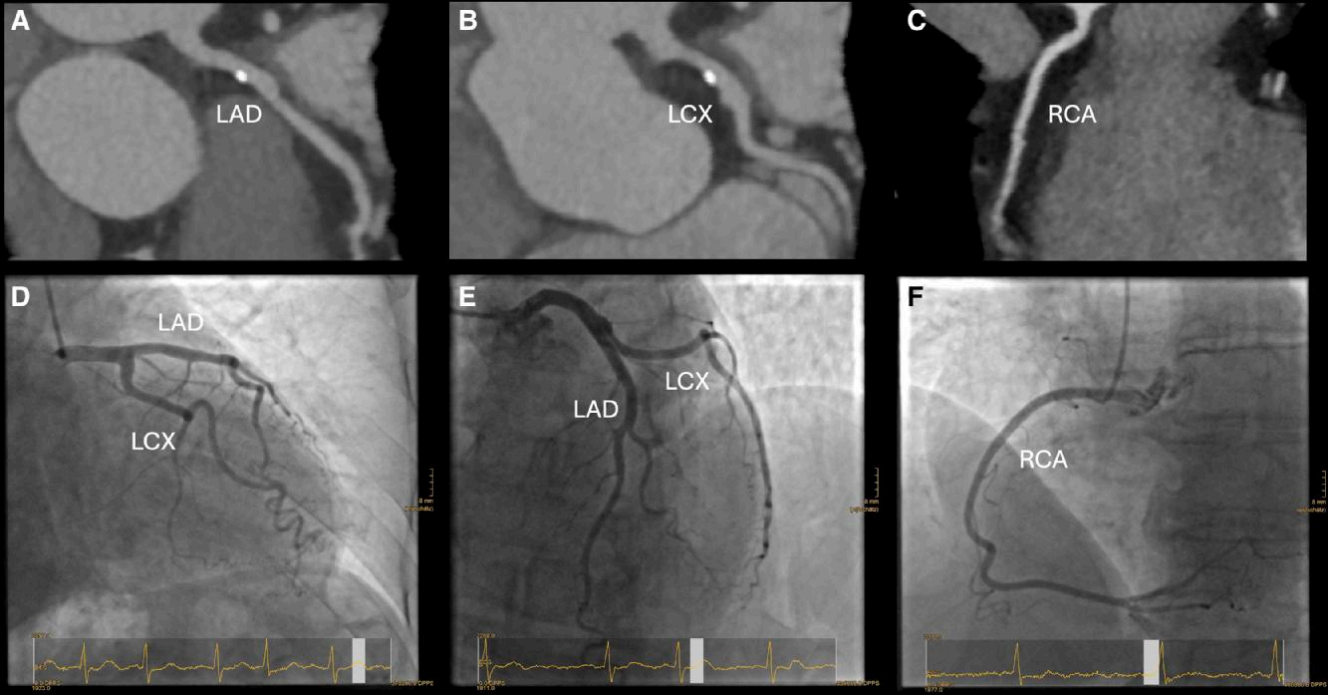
CTA (TAVI planning CT) and corresponding invasive CA and no coronary disease. A 84-year-old female patient, dyspnoea on exertion New York Heart Association (NYHA) III, no angina pectoris, and impaired kidney function. High-gradient AS on echocardiography with a peak velocity of 4.2 m/s. *A* shows the LAD, *B* shows the LCX, and *C* shows the RCA, all from the TAVI planning CT. *D–F* depict the corresponding images of stenosis-free coronaries by invasive CA. CT, computed tomography; LAD, left anterior descending artery; LCX, circumflex artery; RCA, right coronary artery; TAVI, transcatheter aortic valve implantation.
